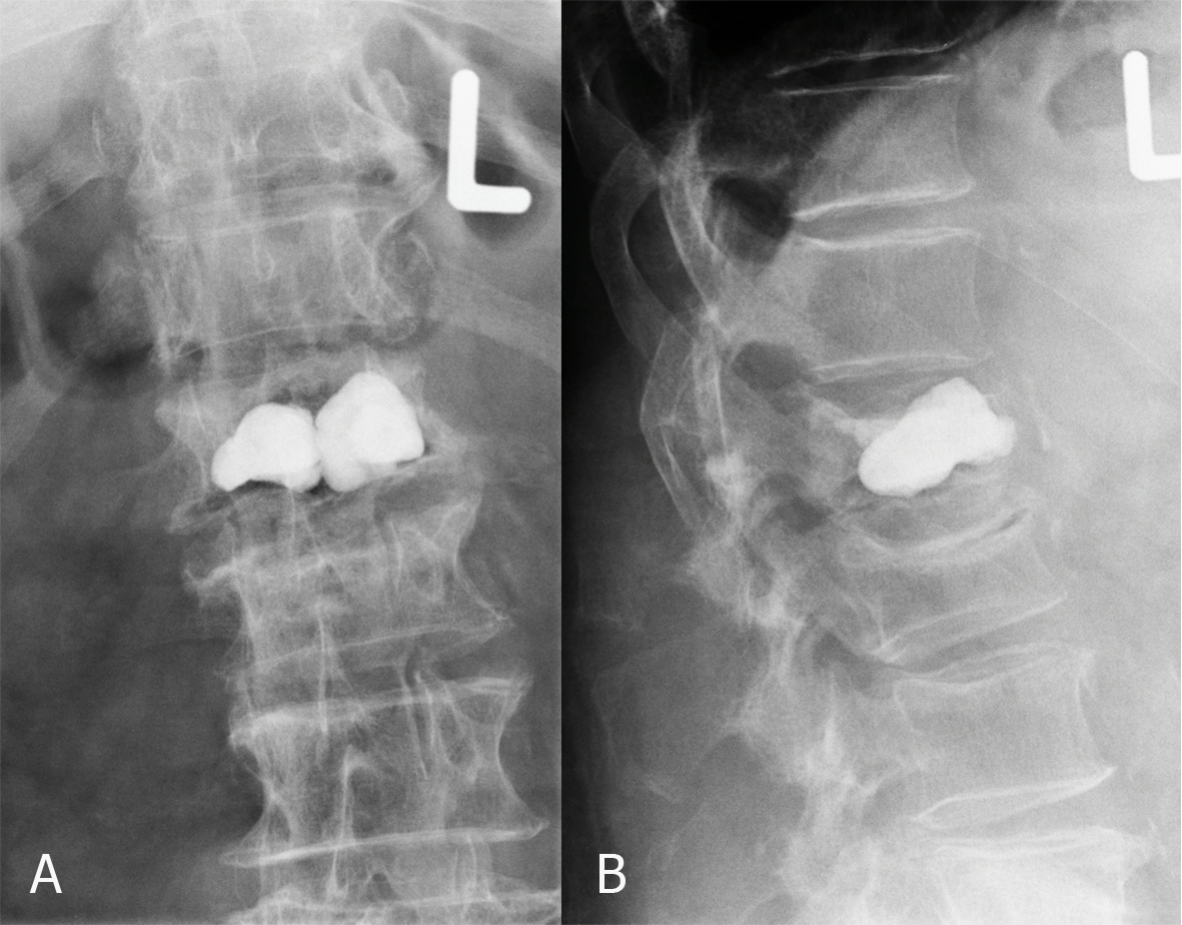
Plain (A) and lateral (B) thoracolumbar radiographs (T11 - L3) taken after initial kyphoplasty for treatment of an L1 compression fracture. The cement is correctly positioned in the vertebral body.
